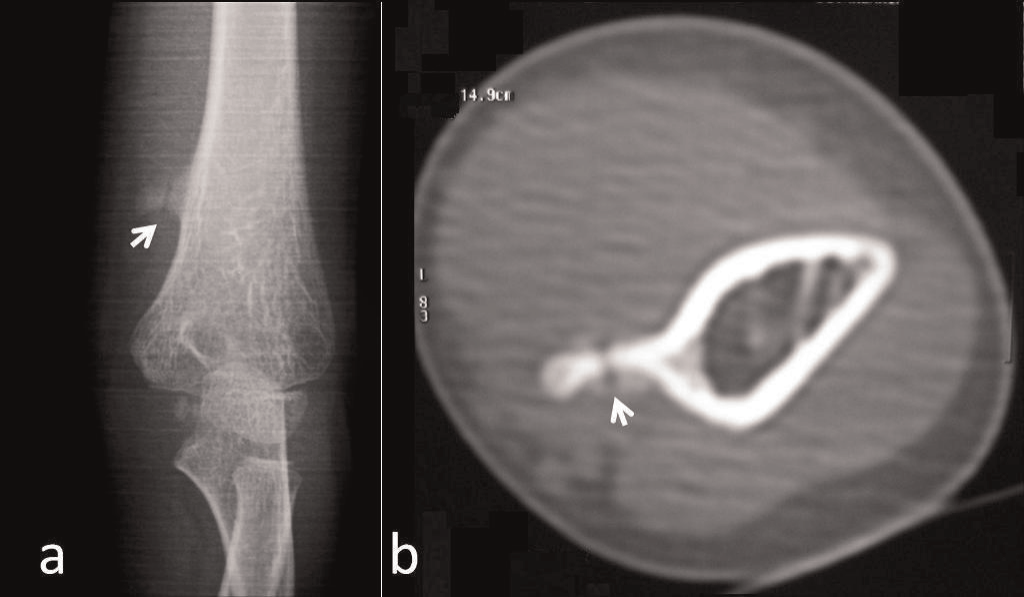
(a) Oblique plain radiograph of the humerus. (b) Axial CT scan of the humerus through the fracture line. White arrows show the fracture.
